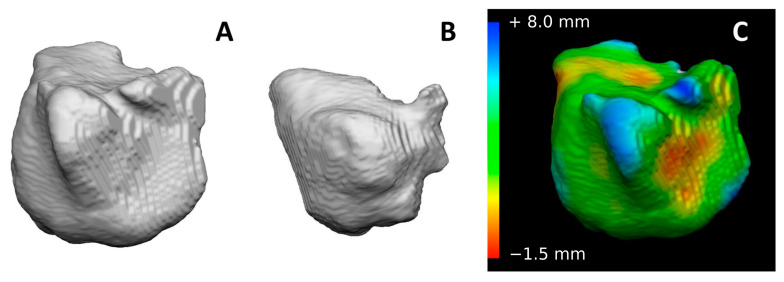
Superimposition of two maxillary sinuses segmented from CT scans belonging to different individuals: (A) AM model (first acquired model) of the first subject, (B) PM model (second acquired model) of the second subject, (C) chromatic visualization of surface differences between the two models according to RMS values: red, yellow and blue colors are predominant and indicate discrepancies between the two superimposed surfaces. On the left side, the legend indicates the point-to-point distance between the two models according to color map.
